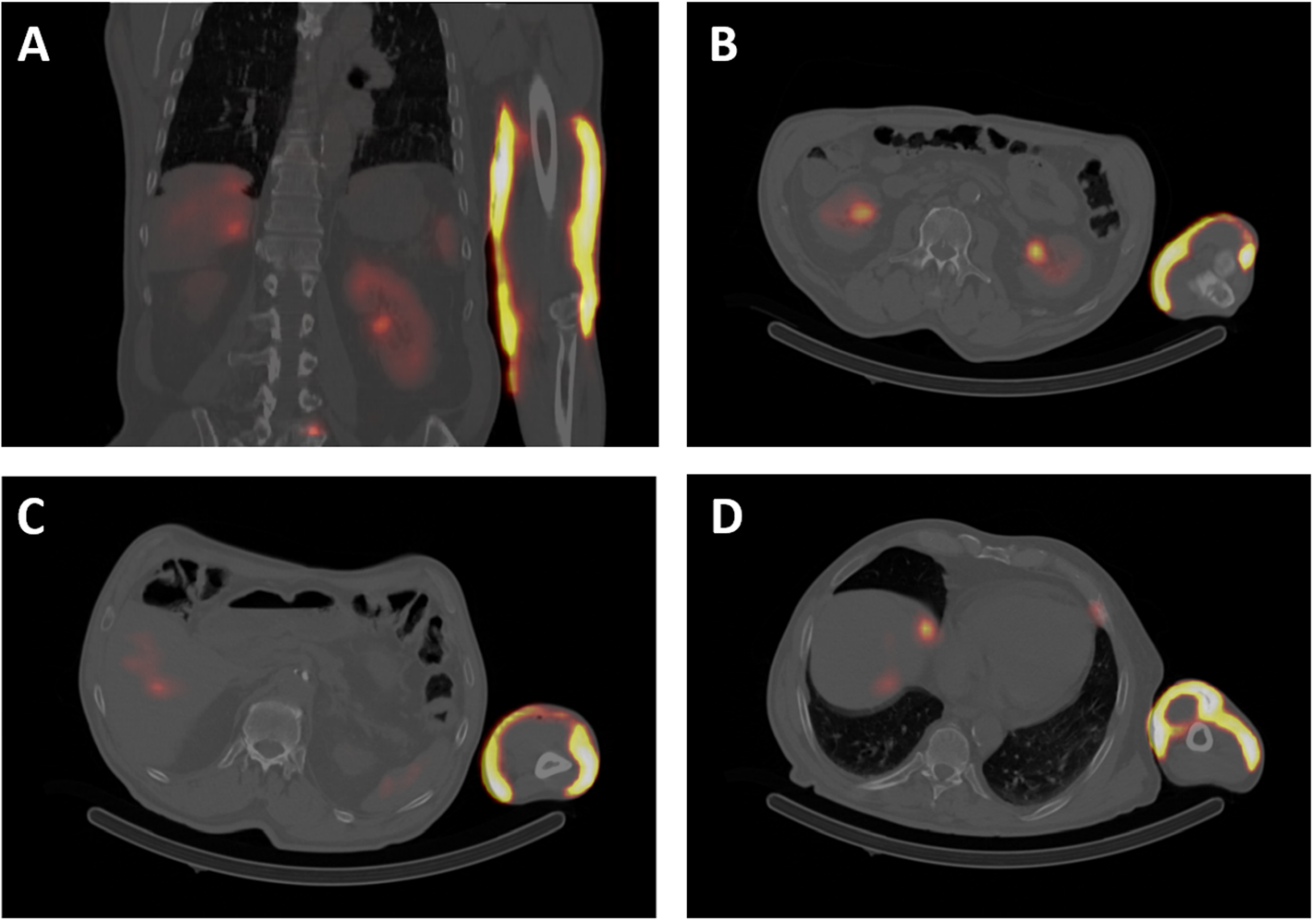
Coronal slice (a) and axial slices (b–d) of *T*_5h_ SPECT/CT showing the subcutaneous distribution of the extravasation in the arm
